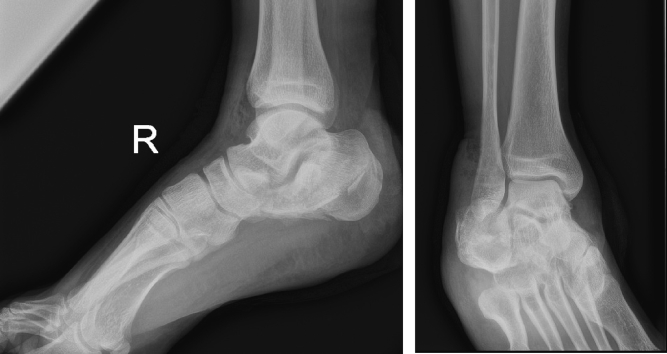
AP + lateral ankle x-ray on presentation to ER.
